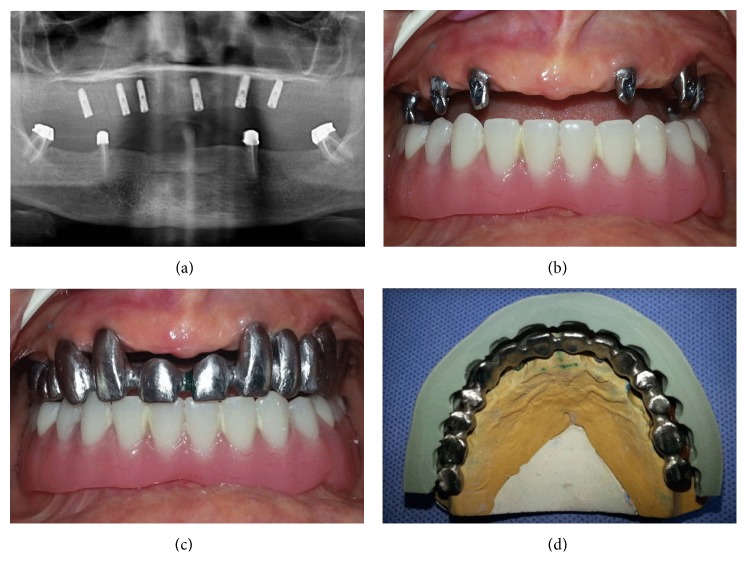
(a) Orthopantomographic view of the case with dental implants. Intraoral view of implant abutments (b) and titanium framework (c). (d) The image of titanium framework with silicone index on the stone model.
